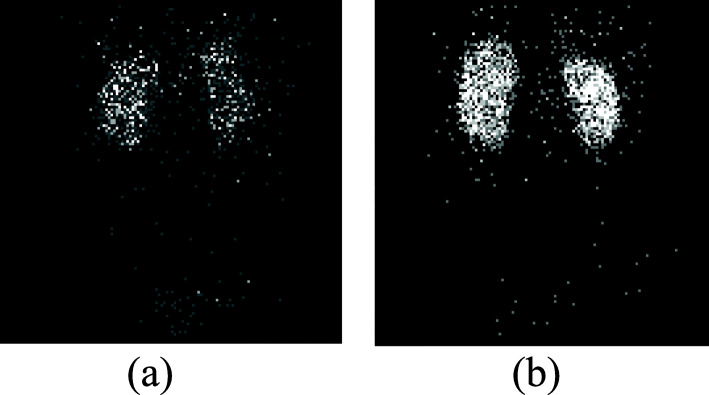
^99m^Tc-DMSA raw projection SPECT images of a representative patient a anterior and b posterior views
